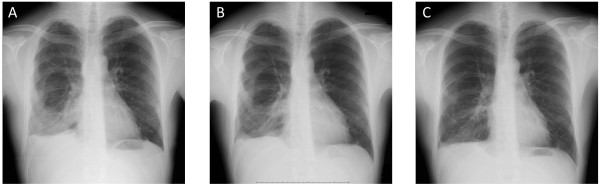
Chest radiograph. A: Pleural based opacities in the right hemithorax at presentation. B: Marked improvements after 6 months of antituberculous chemotherapy. C: Further improvements another 6 months after the completion of chemotherapy.
